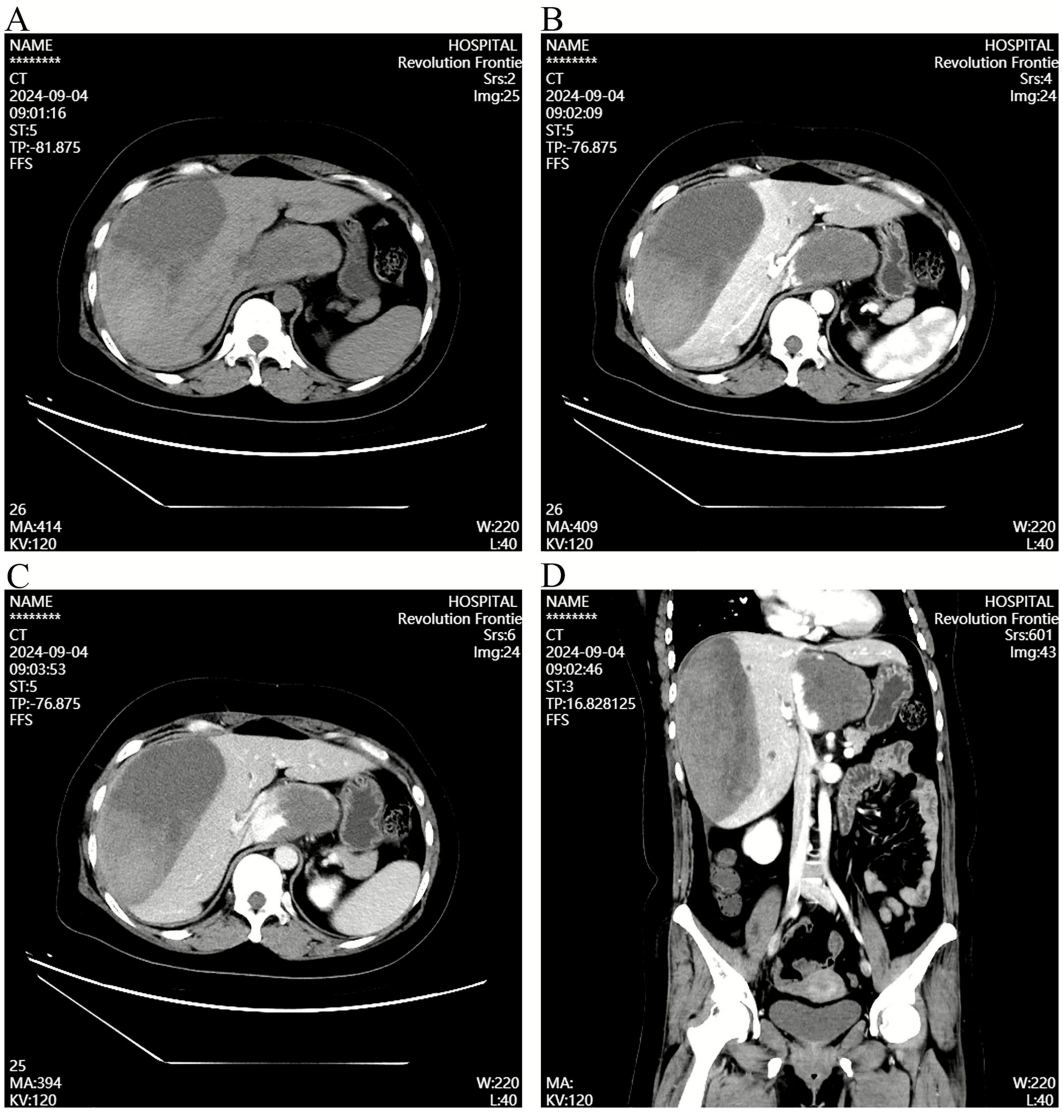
Emergency contrast-enhanced CT revealing subcapsular hematoma and hepatic hemangioma. (A) Unenhanced CT phase showing the subcapsular hematoma and caudate lobe hemangioma. (B) Arterial phase highlighting the subcapsular hematoma and hemangioma. (C) Venous phase depicting the subcapsular hematoma and hemangioma. (D) Coronal reconstruction of contrast-enhanced CT clearly displaying the subcapsular hematoma and caudate lobe hemangioma.
